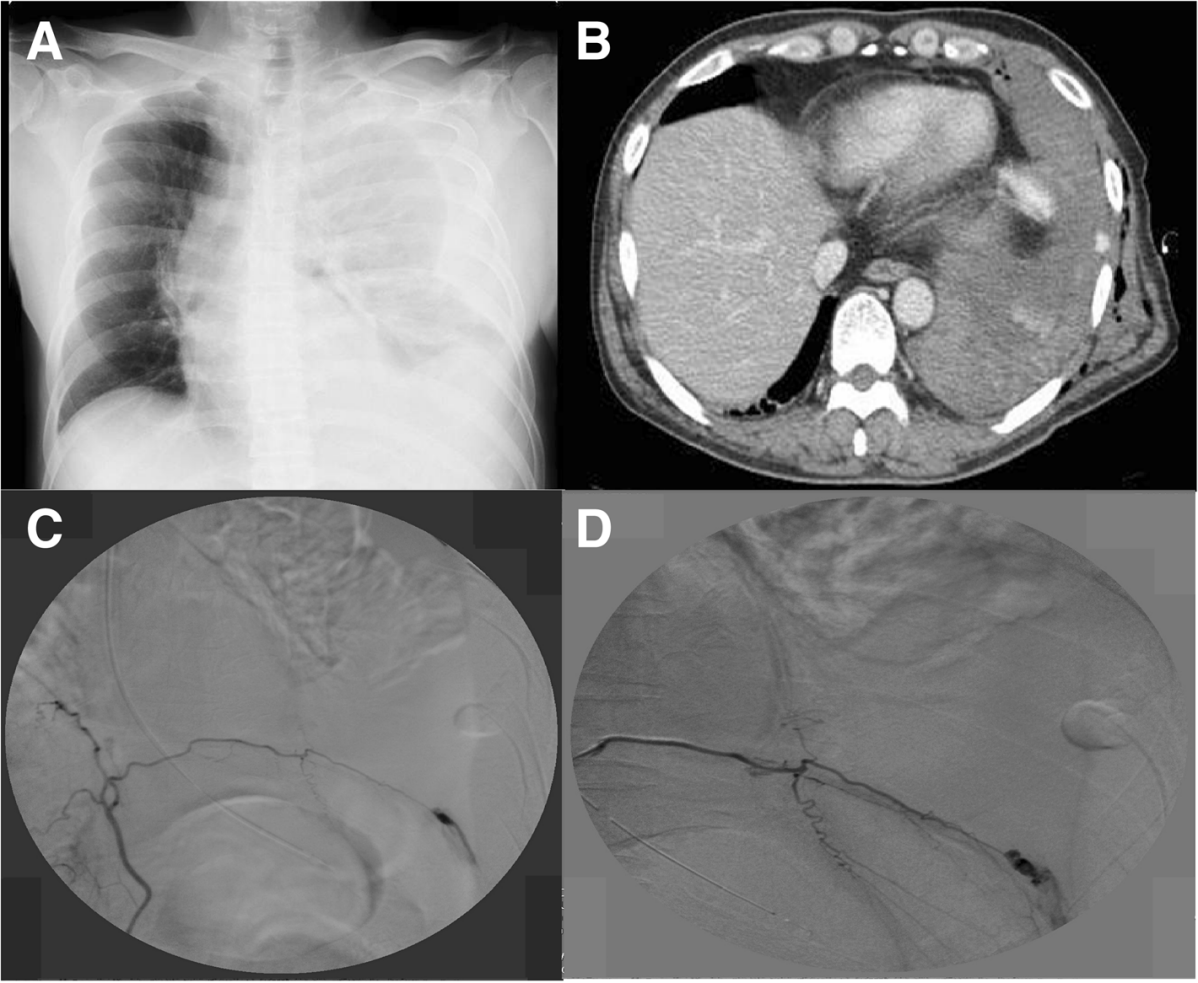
Preoperative images. (A) Chest X-ray film showing left tension hemothorax; (B) Enhanced chest computed tomography (CT) shows left hemothorax and bleeding into the thoracic space from a branch of the left inferior phrenic artery without involvement of the abdomen in the delay phase; (C) Note the leak of contrast medium into the thoracic space on angiography of the selected left phrenic artery; (D) Bleeding was markedly reduced after coil embolization.
